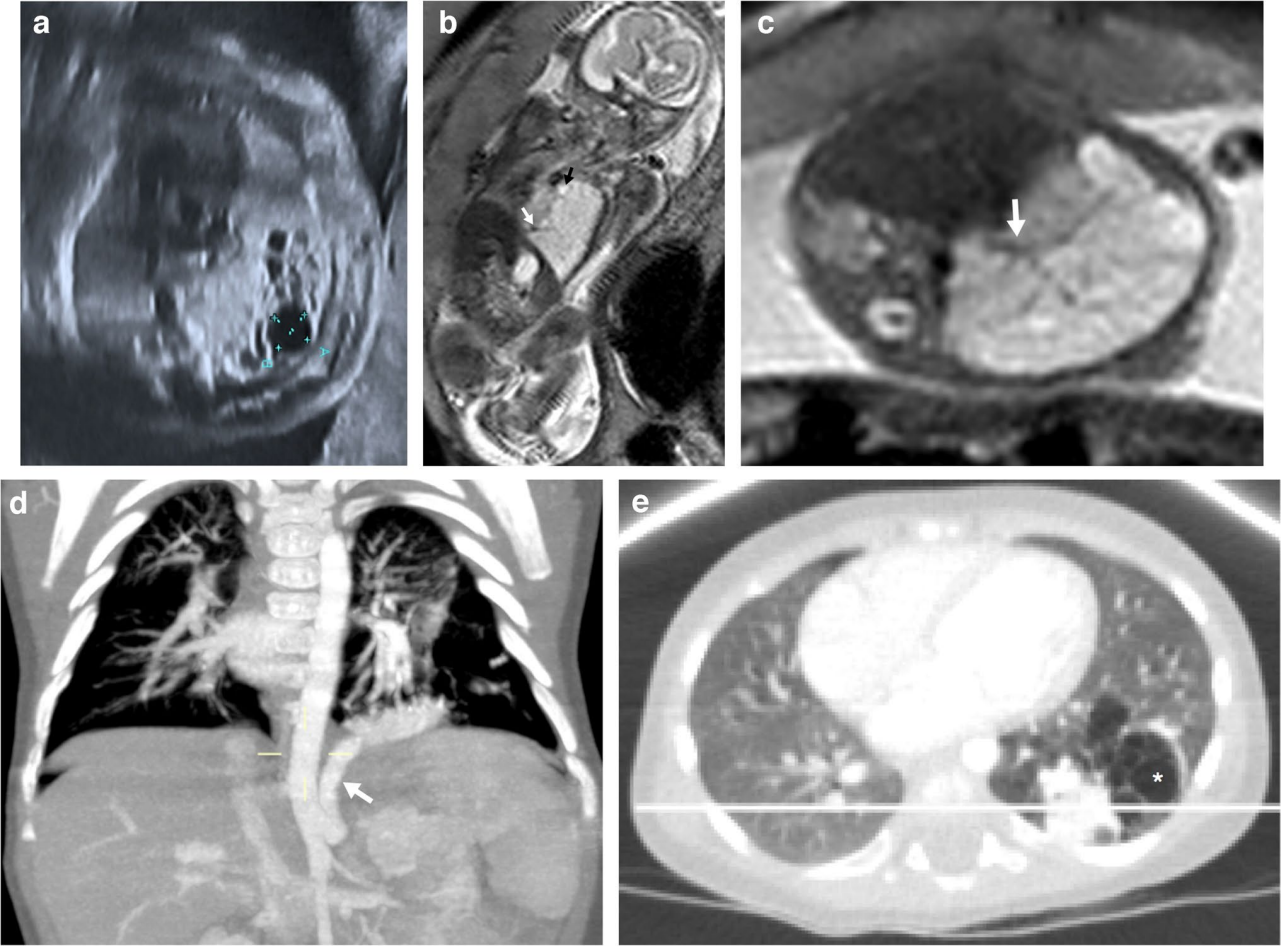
A hybrid lesion (intralobar sequestration and congenital pulmonary airway malformation) in a 21-week gestational age female fetus. a An axial ultrasound image demonstrates a hyperechoic lesion in the left lung base with multiple cysts within it (*calipers*). b, c Fetal magnetic resonance imaging was performed at 22-weeks gestational age. Single-shot T2 coronal (b) and axial (c) images show a large homogeneous lesion in the left lung, with high signal intensity on T2. There is a feeding vessel (*white arrows*) arising from the descending thoracic aorta and small cysts in the periphery of the lung (*black arrow in *b), suggestive of a hybrid lesion. d, e Coronal maximum intensity projection (d) and axial lung window (e) computed tomography images obtained at 5 months of age confirm the lesion in the left lung base and feeding vessel (*arrow* in d) and multiple cysts (*asterisk in *e). Surgery demonstrated a hybrid lesion (intralobar sequestration and congenital pulmonary airway malformation)
